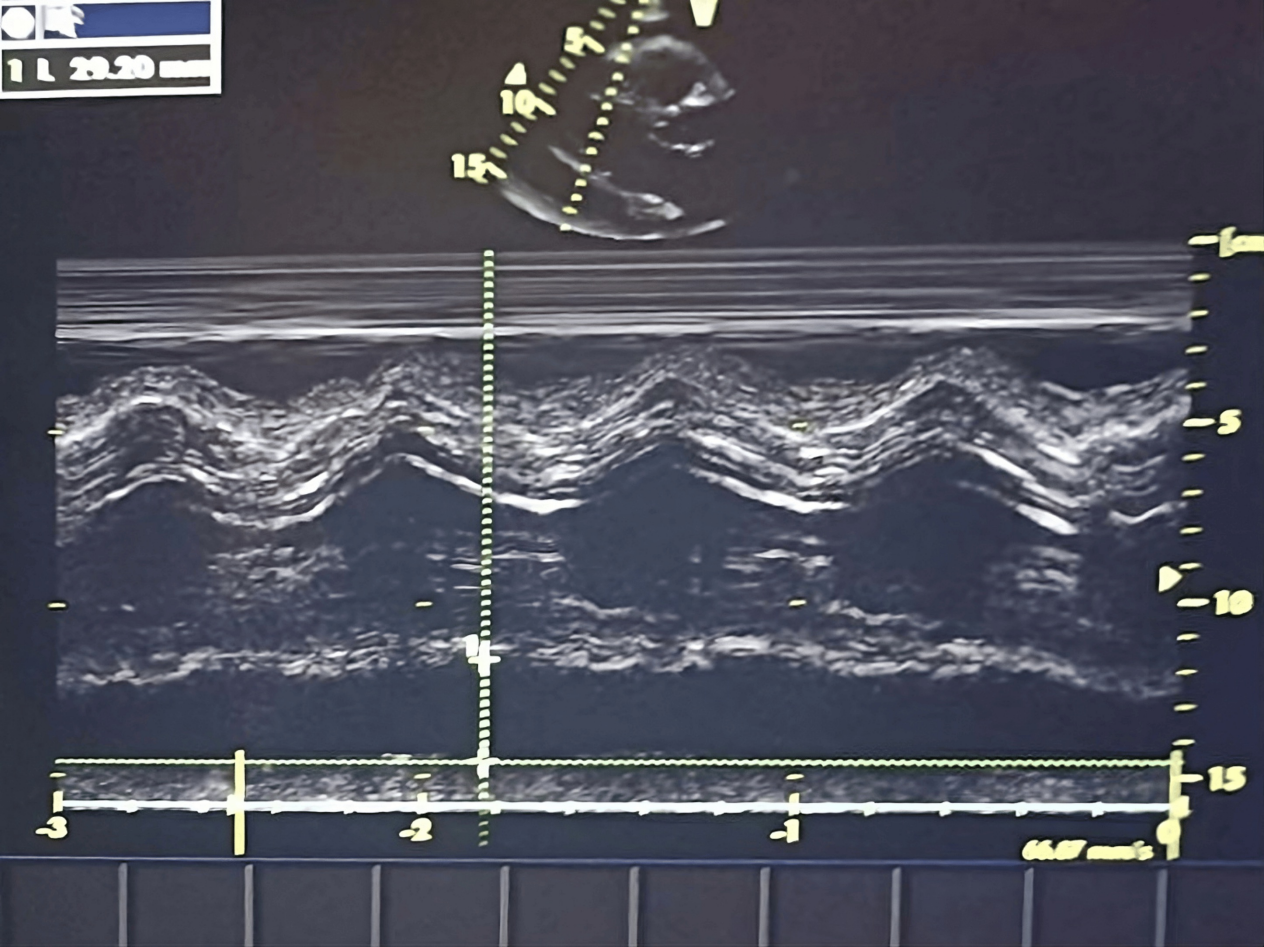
Bedside cardiac ultrasound showing a 29.20 mm posterior pericardial effusion.
